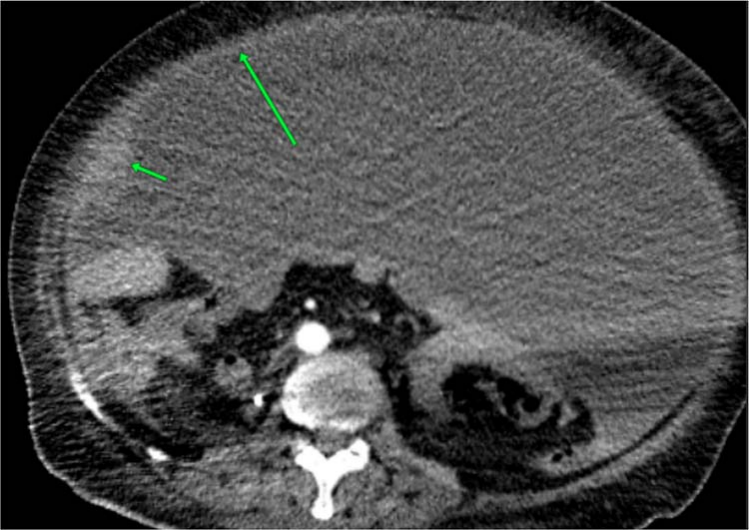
Enhanced computed tomography shows a large volume of ascites with grows and irregular peritoneum circumference.
